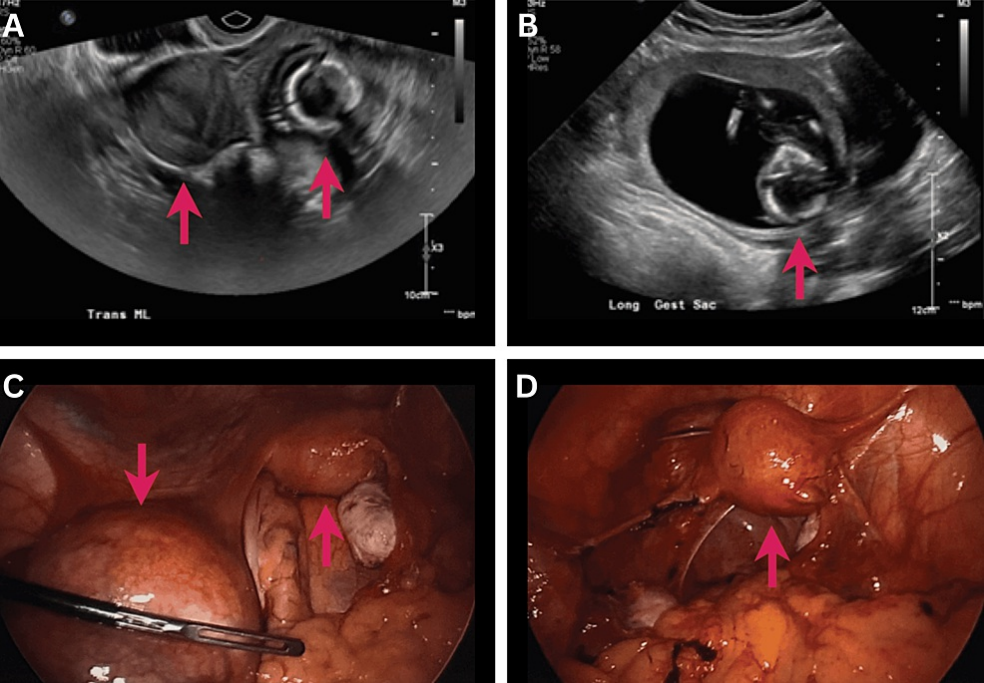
Ultrasound and intraoperative images demonstrating left rudimentary uterine horn pregnancy (A) Transvaginal ultrasound showing an empty dominant uterine horn (left red arrow) and a rudimentary horn containing pregnancy (right red arrow). (B) Transabdominal ultrasound showing fetal calvarium in the left adnexal region (red arrow). (C) Intraoperative visualization of the unicornuate uterus (right red arrow) with the rudimentary horn containing gestation (left red arrow, in contact with the Dorsey grasper) before excision. (D) Laparoscopic image of the remaining dominant horn (red arrow) after rudimentary horn excision.
